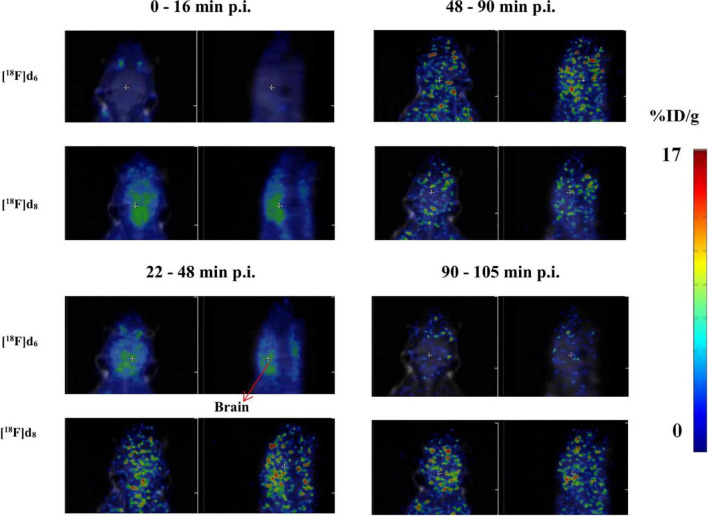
Representative axial and sagittal images of the brain accumulation of [^18^F]d_6_ and [^18^F]d_8_ displayed as SUVs.
